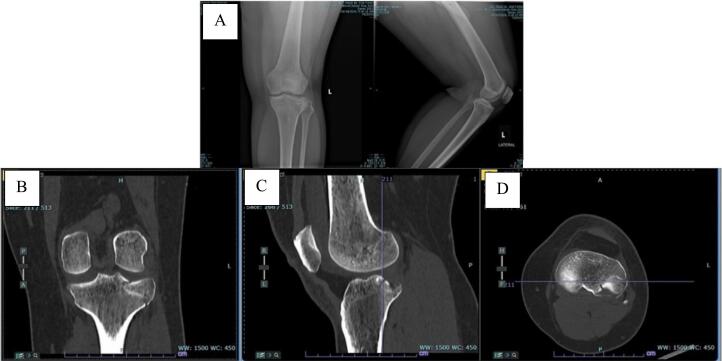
This Picture shows Xray and CT-Scan of left knee before performing surgery. The picture shows knee from some position. A) Xray AP/Lat of the Left Knee, B) Coronal CT Scan, C) Sagittal CT Scan, and D) Axial CT axial.
